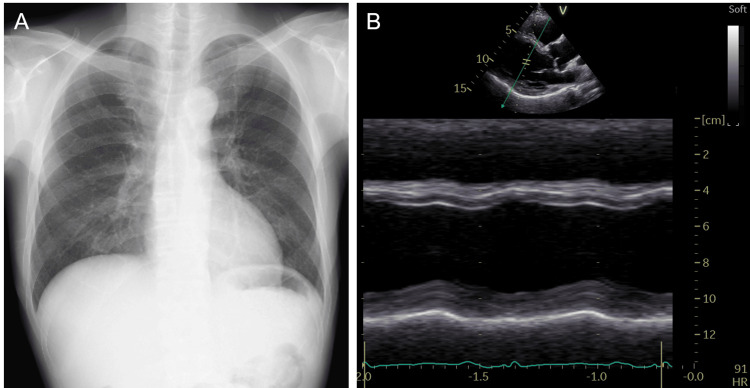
Chest radiography (A) and echocardiography (B) at discharge
